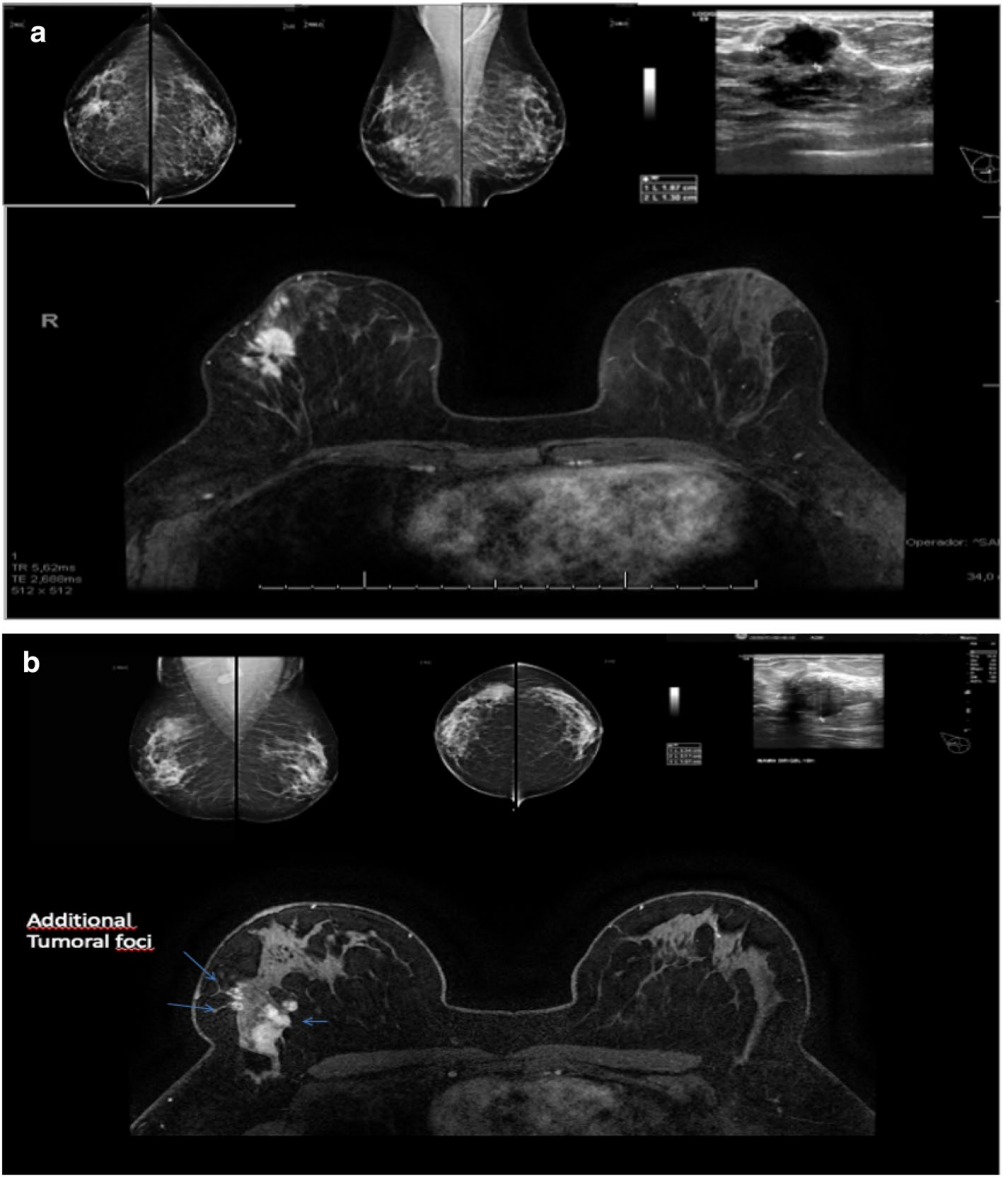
MRI additional findings. a Mastectomy due to a tumor 50% larger than evaluated by mammography and/or ultrasound and the breast still allows a conservative surgery. Mammography report (MMG): Hyperdense, irregular, and spiculated nodule, associated with tenuous amorphous calcifications, located in the lower outer quadrant (LOQ) of the right breast, measuring 1.8 × 2.8 cm. Corresponds to the irregular nodule in ultrasound are situated in the LOQ of the right breast, 1.9 × 1.3 × 1.3 cm. MRI report: Irregular nodule with spiculated margin, no signs of cutaneous involvement, located in the middle third of the LOQ of the right breast. Measures 3.0 × 3.0 × 2.0 cm. b Multicentricity tumor in MRI exam undetected by other methods. MMG report: focal asymmetry associated with round calcifications located in the right breast upper outer quadrant (UOQ), in agreement with an irregular nodule characterized at ultrasound in the right breast UOQ with 2.7 × 2.5 × 2.1 cm. The MRI report showed an irregular nodule with heterogeneous internal enhancement and progressive kinetic curve, placed in the posterior third of the UOQ of the right breast, measuring 4 × 3.4 × 2.7 cm. Associated with a focal clumped type enhancement with a progressive kinetic curve, with an extension of 3.1 cm anterior to it, which together measure 5.6 cm
